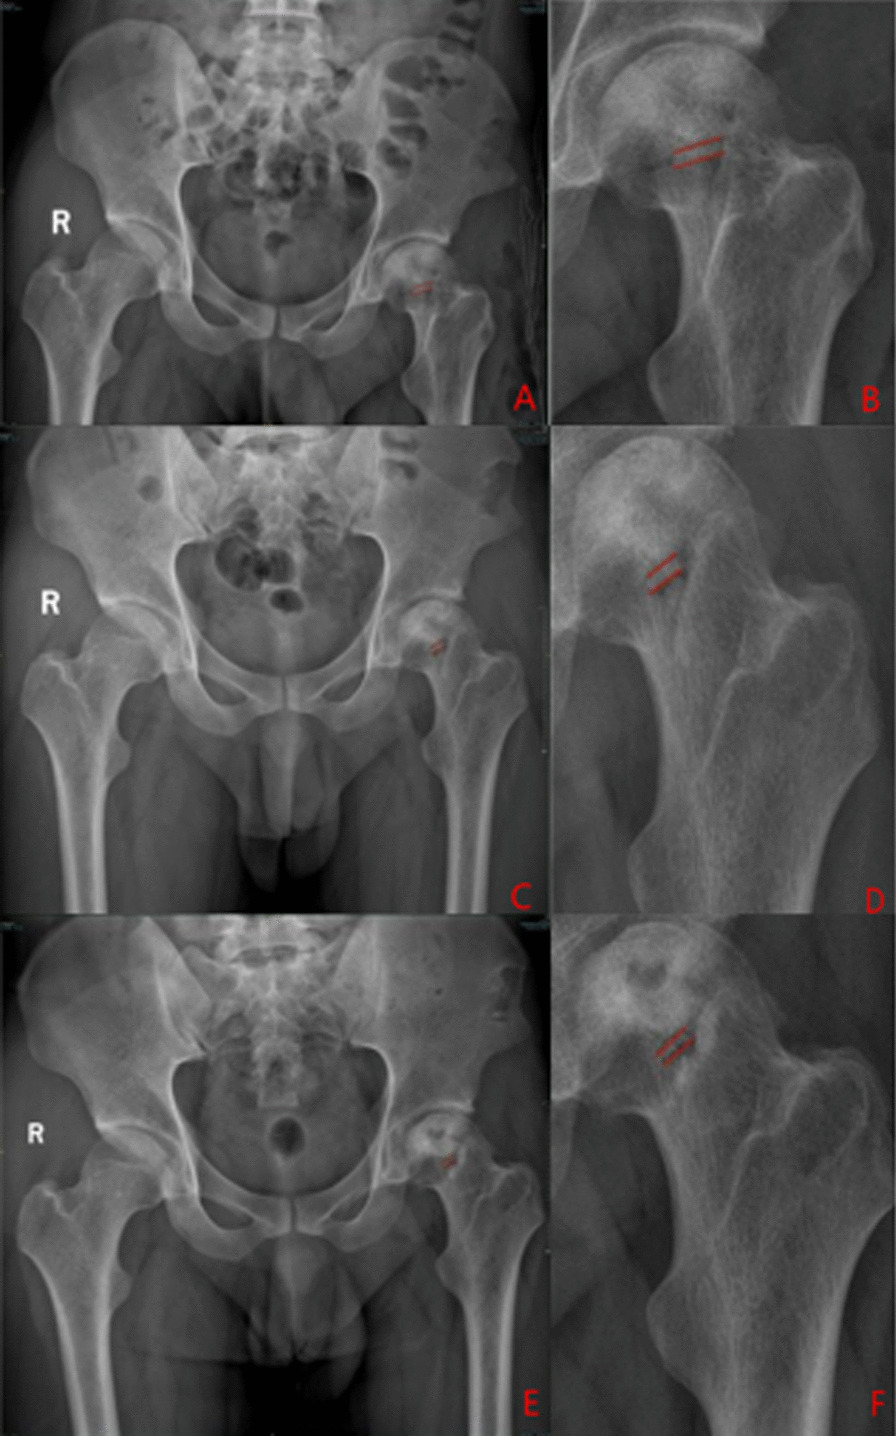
Post-operative (A, B), 3-month (C, D) and 6-month post-operative (E, F) radiographs of a typical patient showing changes in the size of biodegradable magnesium screw
